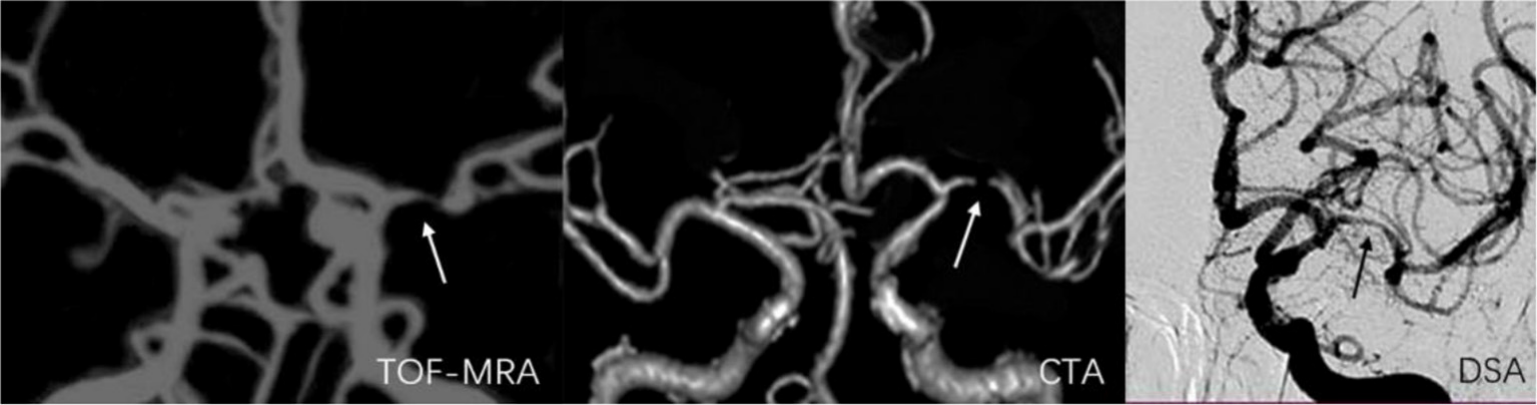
A 55-year-old man with stenosis on the bifurcation and bend of the left middle artery. The arrow marks the location of the lesion. The degree of stenosis acquired on TOF-MRA (75.3%) and CTA (92.5%) exceeded that on DSA (56.9%).
